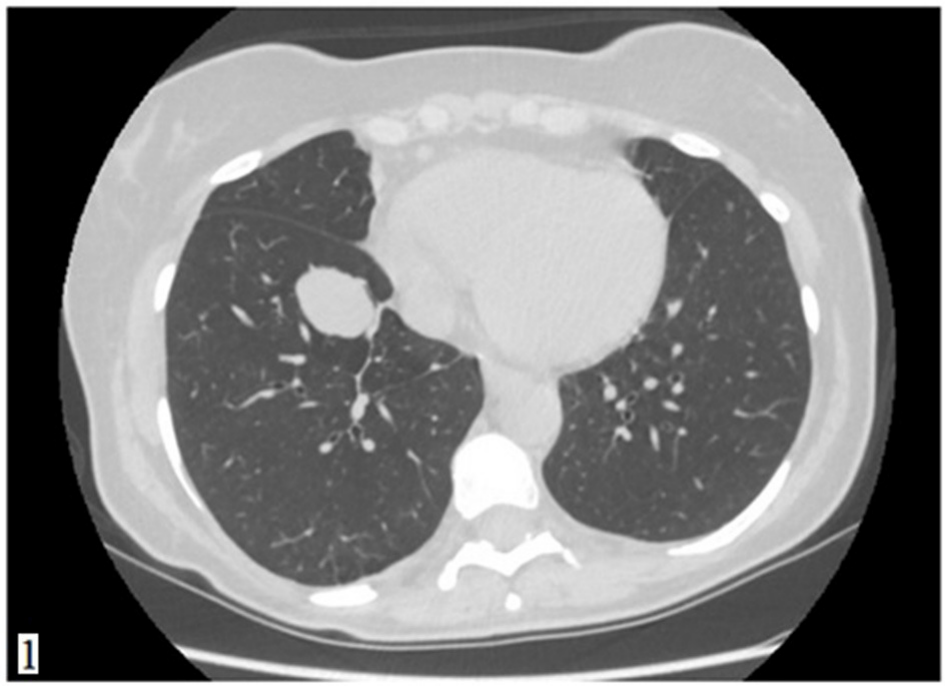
A CT scan showing a solid lesion with well-defined margins in the anterior segment of the inferior lobe of the right lung.
